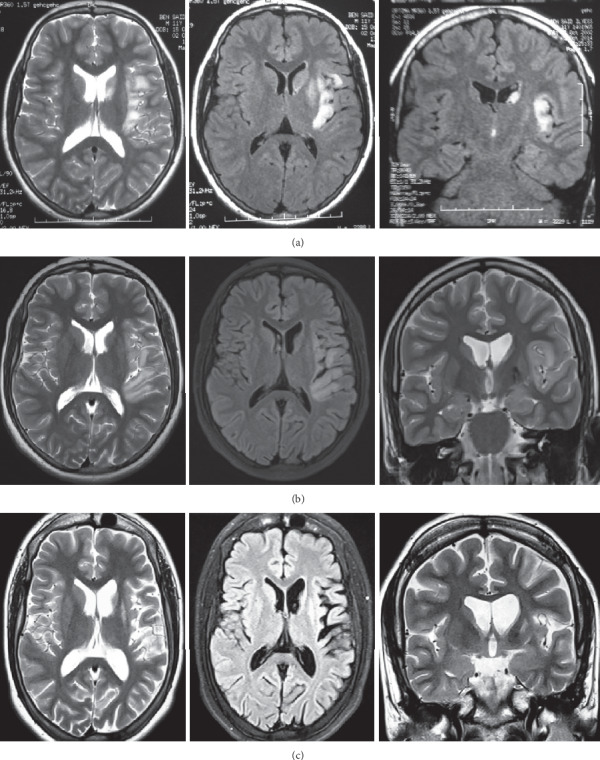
Serial MRIs in T2 and FLAIR sequences showing hyperintensity in the left frontoinsular region, lenticular, and caudate nuclei (a). Seven months after the first MRI, persistence of hyperintensity, left fronto-insular cortical and homolateral striatum atrophy, and dilatation of the ipsilateral ventricular system (b). After 3 years and 9 months from the first MRI, we noticed an increase of the left hemispheric atrophy (c).
